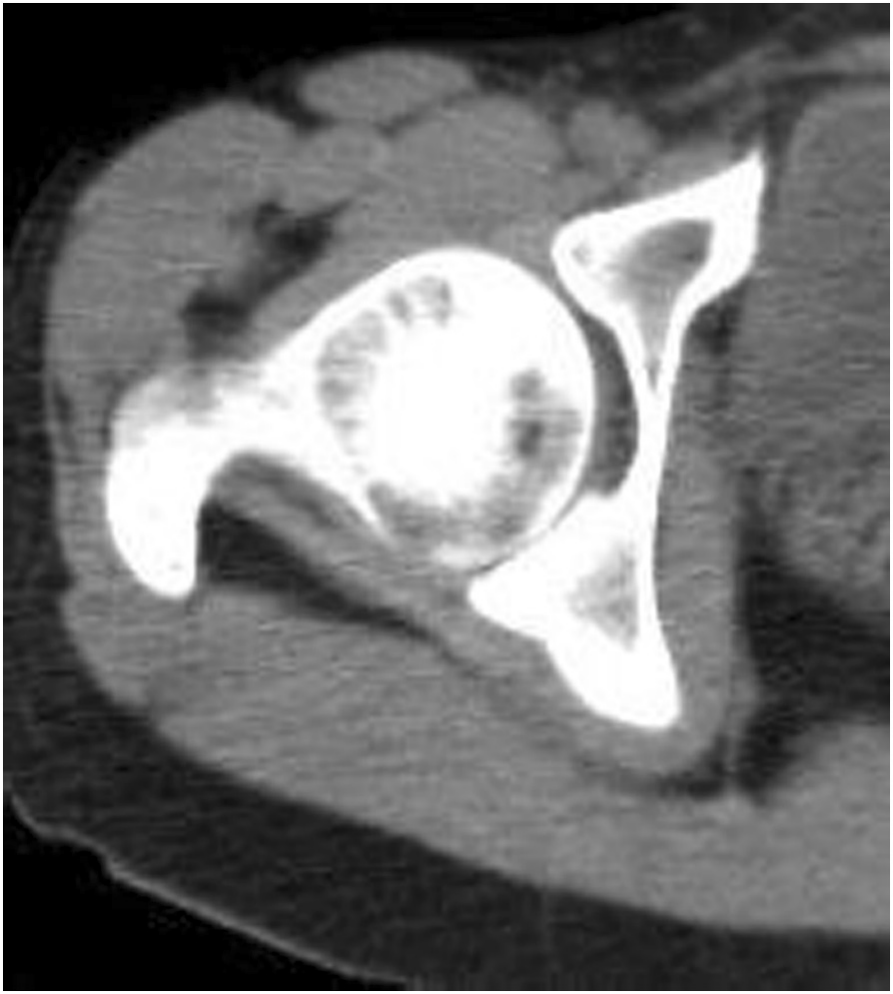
Computed tomography: the α angle was 50°, and no cam lesion was observed.
